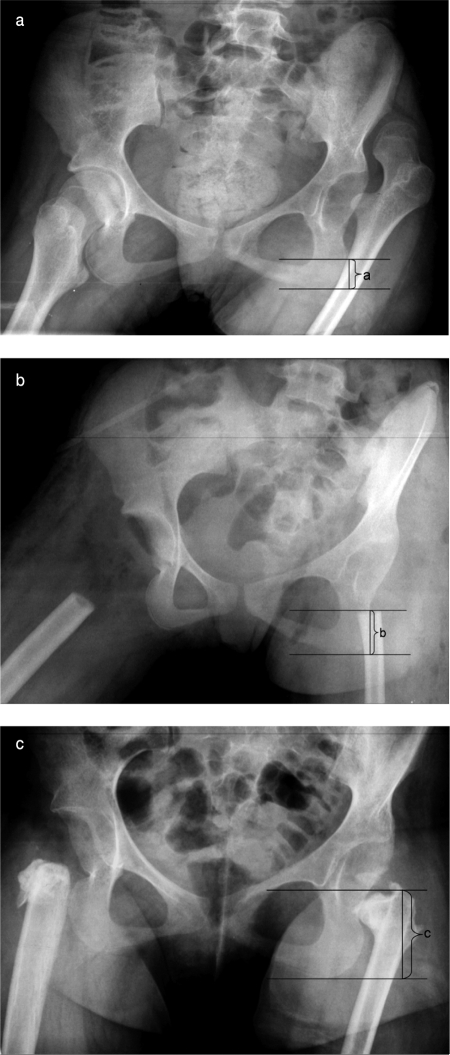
Patient number 7, a 14-year-old girl with quadriplegic cerebral palsy. She had severe pain, was unable to sit in her wheelchair, and had problems with perineal care. A. Radiograph showing left hip dislocation indicating the vertical distance (a) from the femoral resection level to the distal horizontal tangent of the os ischii. B. Radiograph 1 day postoperatively after bilateral PFRIA, showing the vertical distance (b) from the proximal femur to the distal horizontal tangent of the os ischii. C. Radiograph at 16 months, showing the vertical distance (c) from the proximal femur to the distal horizontal tangent of the os ischii and showing bilateral heterotopic ossification.
